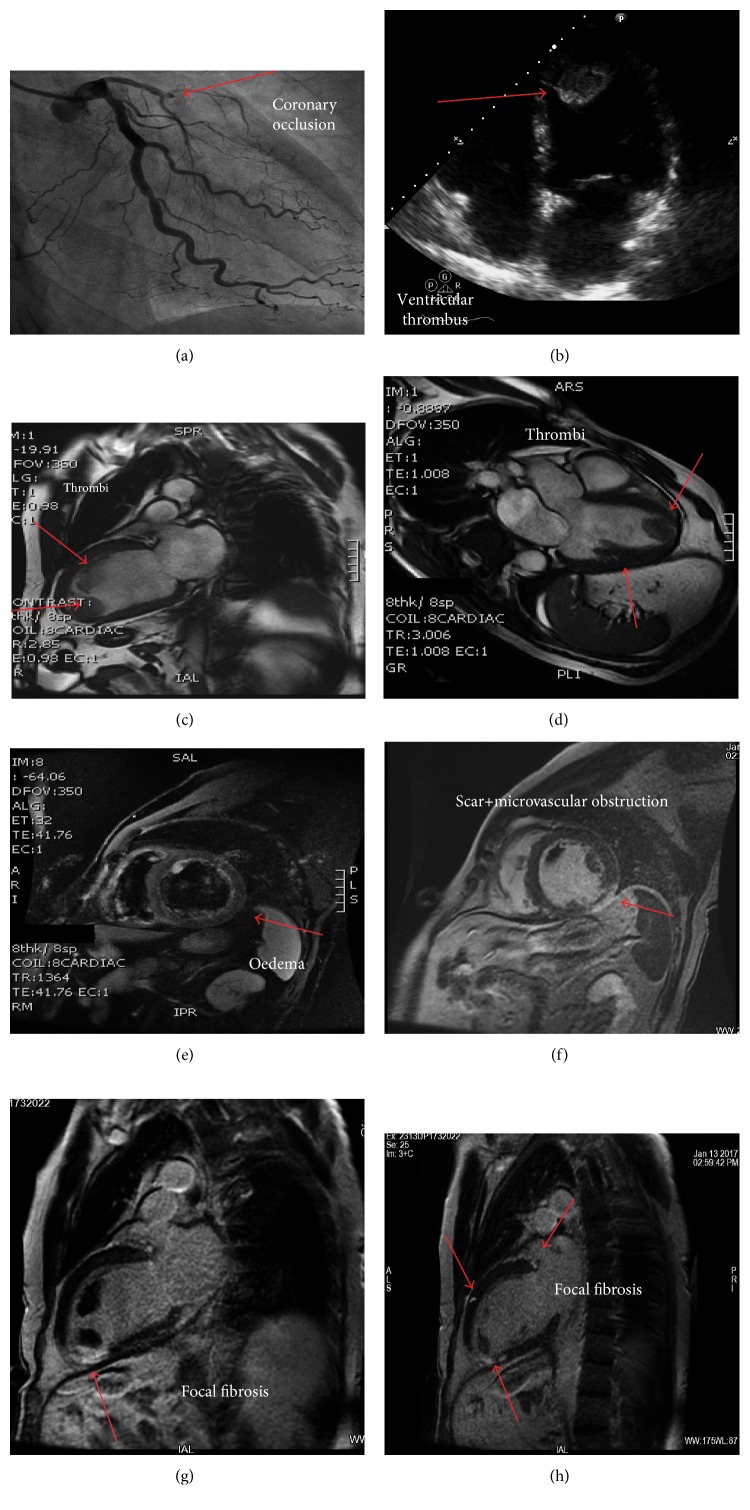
(a) Coronary angiography showing the occlusion of the left descending artery in the proximal segment (arrow); (b) transthoracic 2-D echocardiography showing an apical ventricular thrombus; (c, d) cardiac magnetic resonance (CMR) unveiling the presence of two large left ventricular thrombi in the apex and along the anterior wall; (e) CMR TIR-T2 sequences showing myocardial edema involving the anterior wall of the left ventricle; (f, g) delay enhancement revealing scar, microvascular obstruction, and fibrosis (arrows); (h) CMR imaging: three hyperenhancement focal areas of fibrosis.
